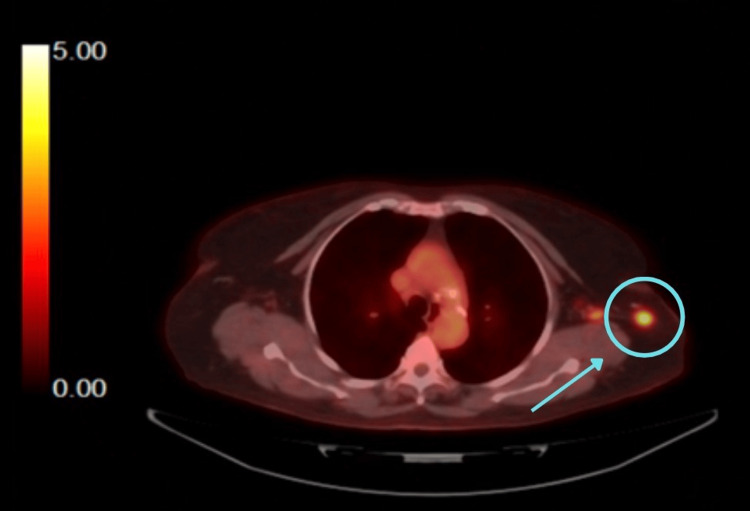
Transverse view of the PET/CT scan showing an FDG-avid left axillary node (standardised uptake value (SUV) 5.8) FDG: Fluorodeoxyglucose
